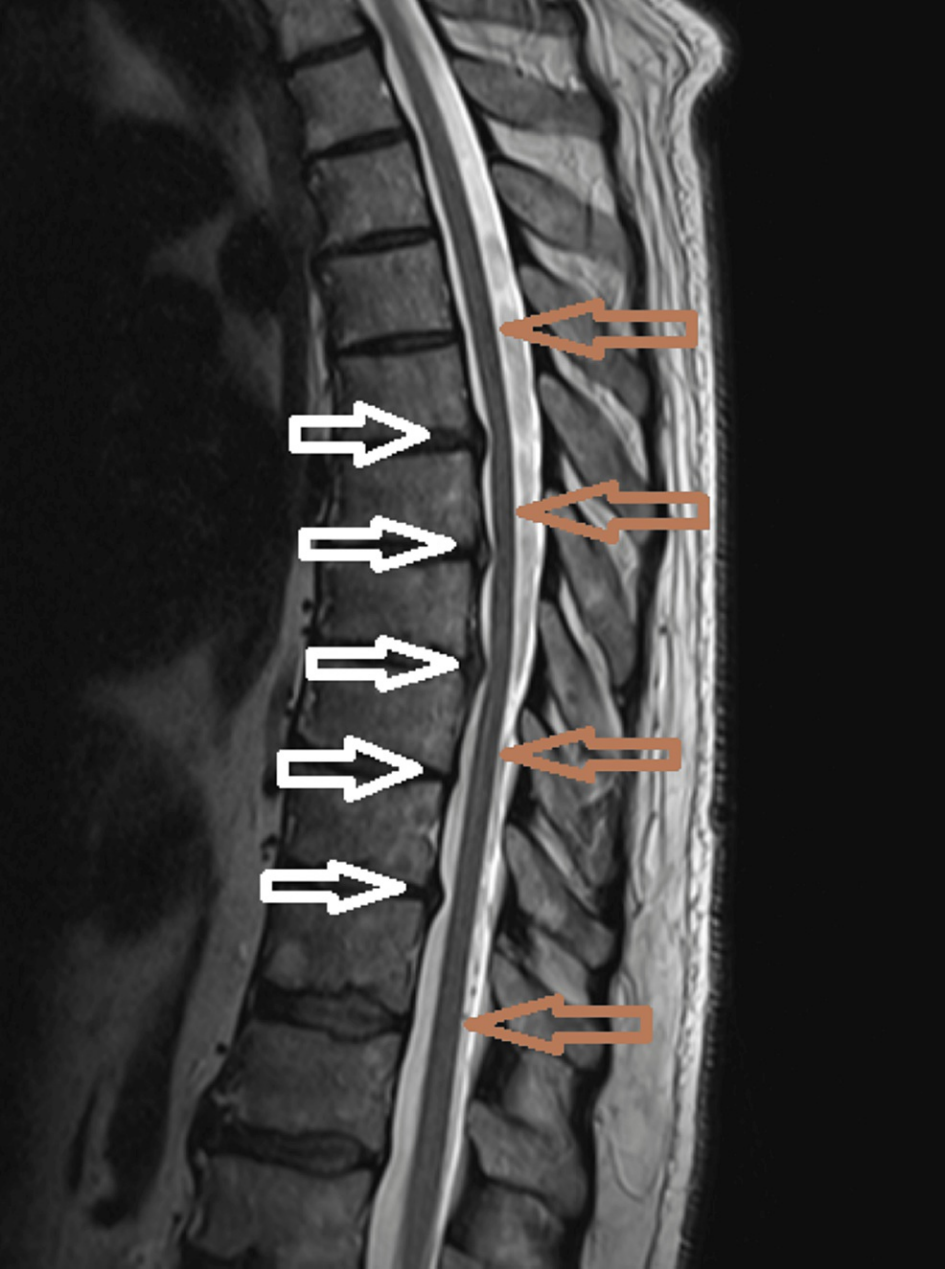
MRI thoracic spine, sagittal view, T2-weighted image, showing disc protrusions (white arrows), without cord compression and normal cord (brown arrows), with no signal changes. MRI - magnetic resonance imaging
